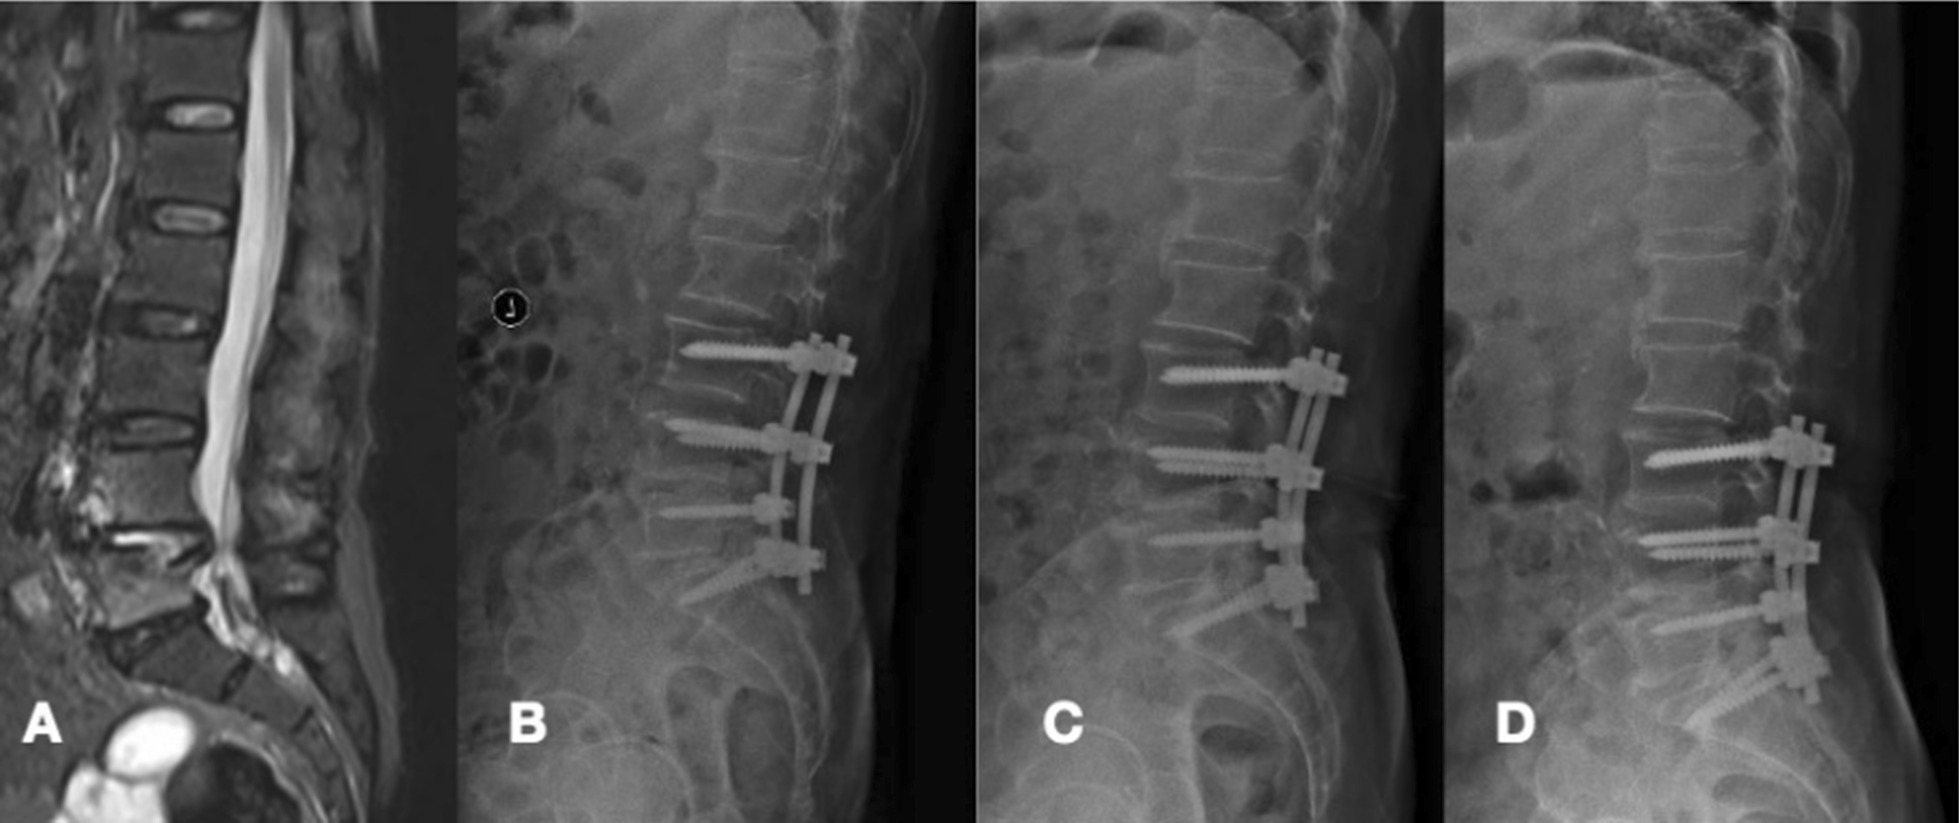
A patient aged 40–50 years old with lumbar pyogenic discitis (L4–5) underwent posterior lumbar interbody fusion combined with instrumentation with instrumentation with an IG. A Preoperative computed tomography (MRI) showed bone destruction of the L4–5 disc and compression of the spinal nerves. B, C Six-month and 12-month postoperative X-rays showed maintained correction. D Three-year postoperative X-rays showed that solid bone fusion had been achieved
